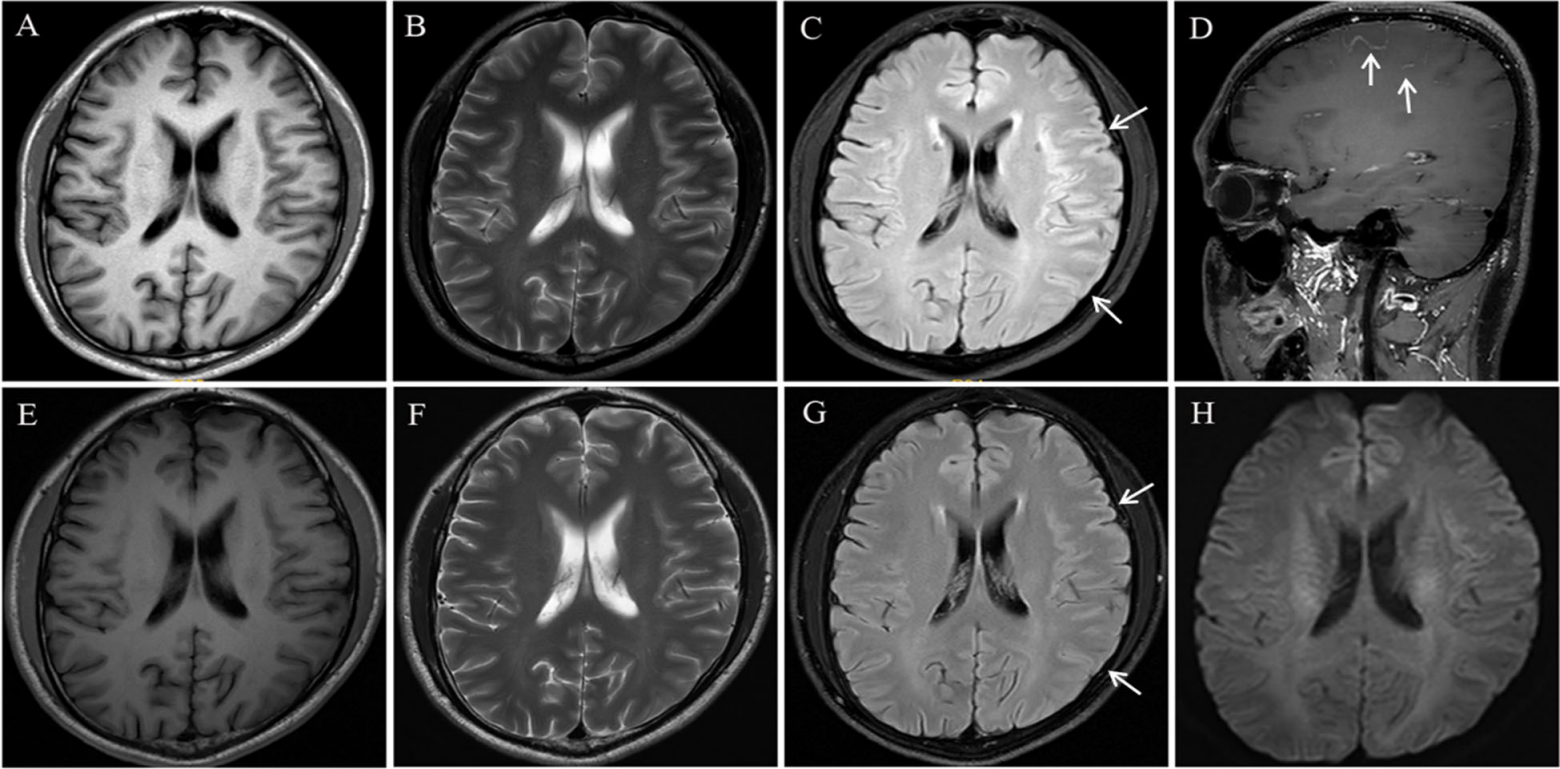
Brain magnetic resonance imaging (MRI): On admission, brain MRI showed no significant signal abnormalities on T1- and T2-weighted sequences (A, B). FLAIR images revealed subtle hyperintensity signal in the right frontoparietal cortex and subcortex, accompanied by gyral swelling and sulcal effacement (C). Post-contrast T1-weighted imaging demonstrated focal leptomeningeal enhancement within the affected region (D). Repeat brain MRI performed at 5-week follow-up demonstrated no abnormal signal on T1- and T2-weighted sequences (E, F), along with significant improvement in FLAIR signal abnormalities accompanied by marked resolution of gyral swelling and sulcal effacement in the frontoparietal regions (G). No abnormal signal was observed on DWI (H).
